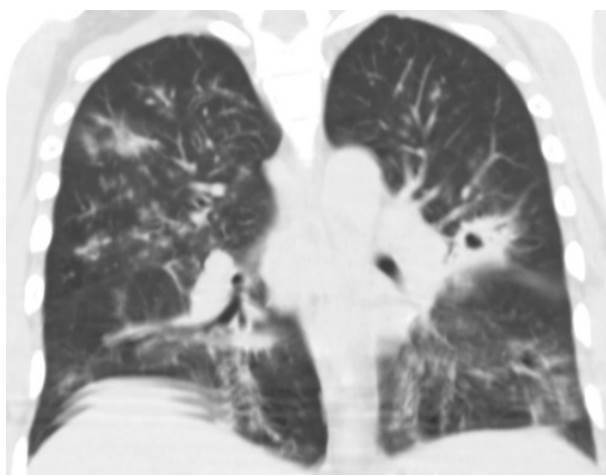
Chest computed tomography, without contrast enhancement, with ground glass lesions (compatible with COVID-19) and a cavitating nodule (compatible with pulmonary cryptococcosis).
